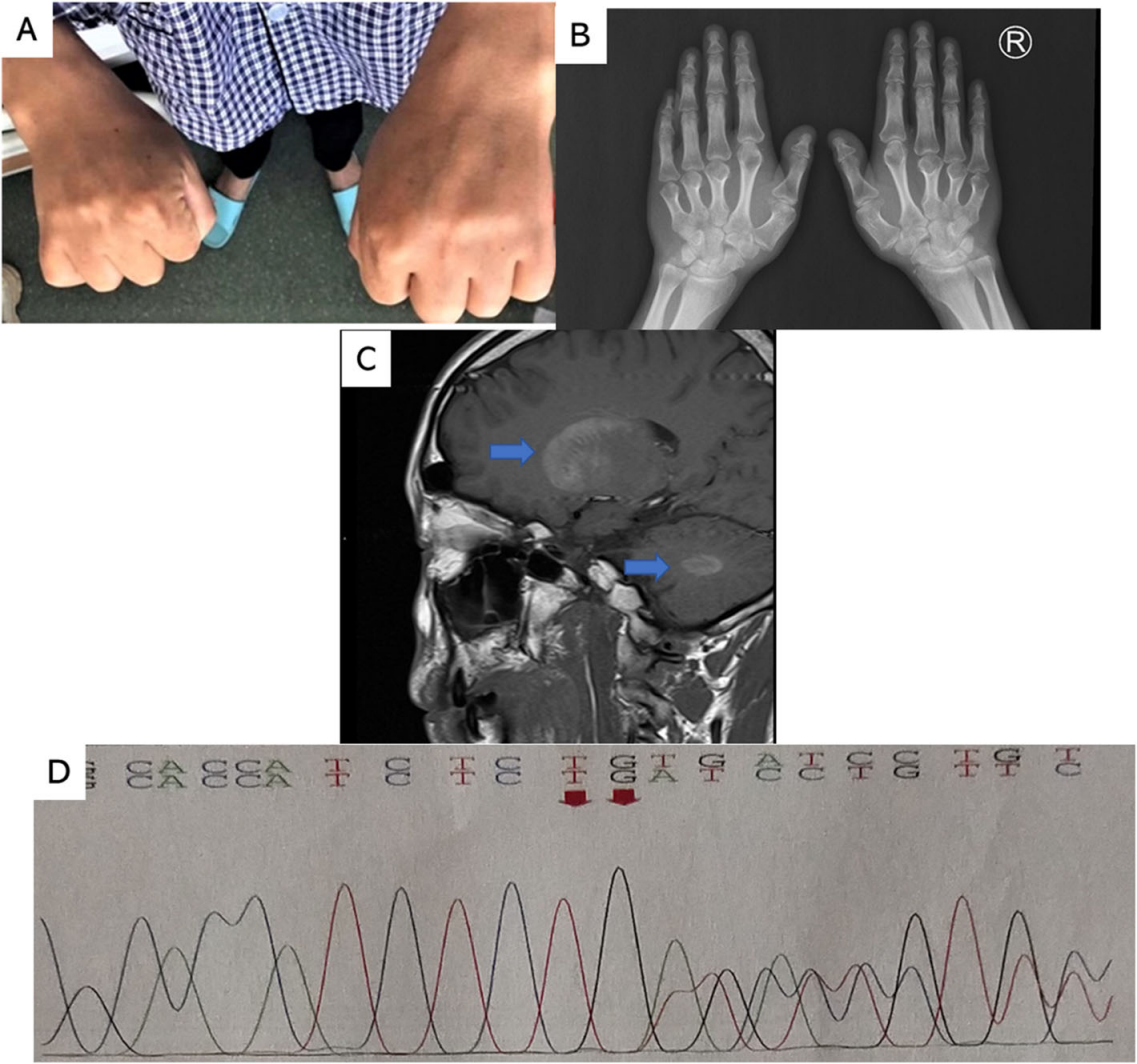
a Brachydactyly in bilateral 1st, 3rd, 4th and 5th fingers of the patient as one of the notable signs of AHO. b Hands X-ray of the patient showing brachydactyly in bilateral 1st, 3rd, 4th and 5th fingers. c Pituitary MRI revealed heterotopic calcification in basal ganglia and cerebellum as pointed by arrows. d A novel frameshift mutation in *GNAS* gene of the patient
